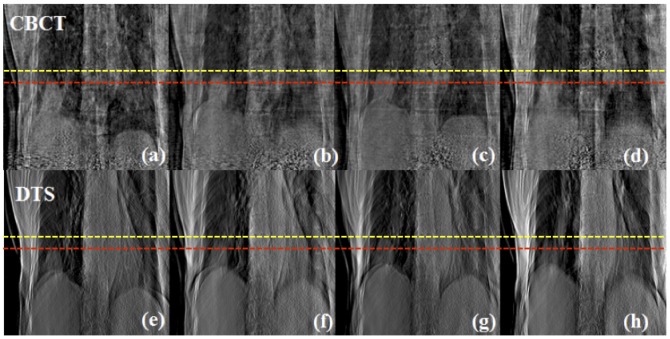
Coronal view of 4D CBCT and DTS reconstructed using lung cancer patient #1 scans with peak-exhale (a), (e), mid-inhale (b), (f), peak-inhale (c), (g) and mid-exhale (d), (h) phases.
